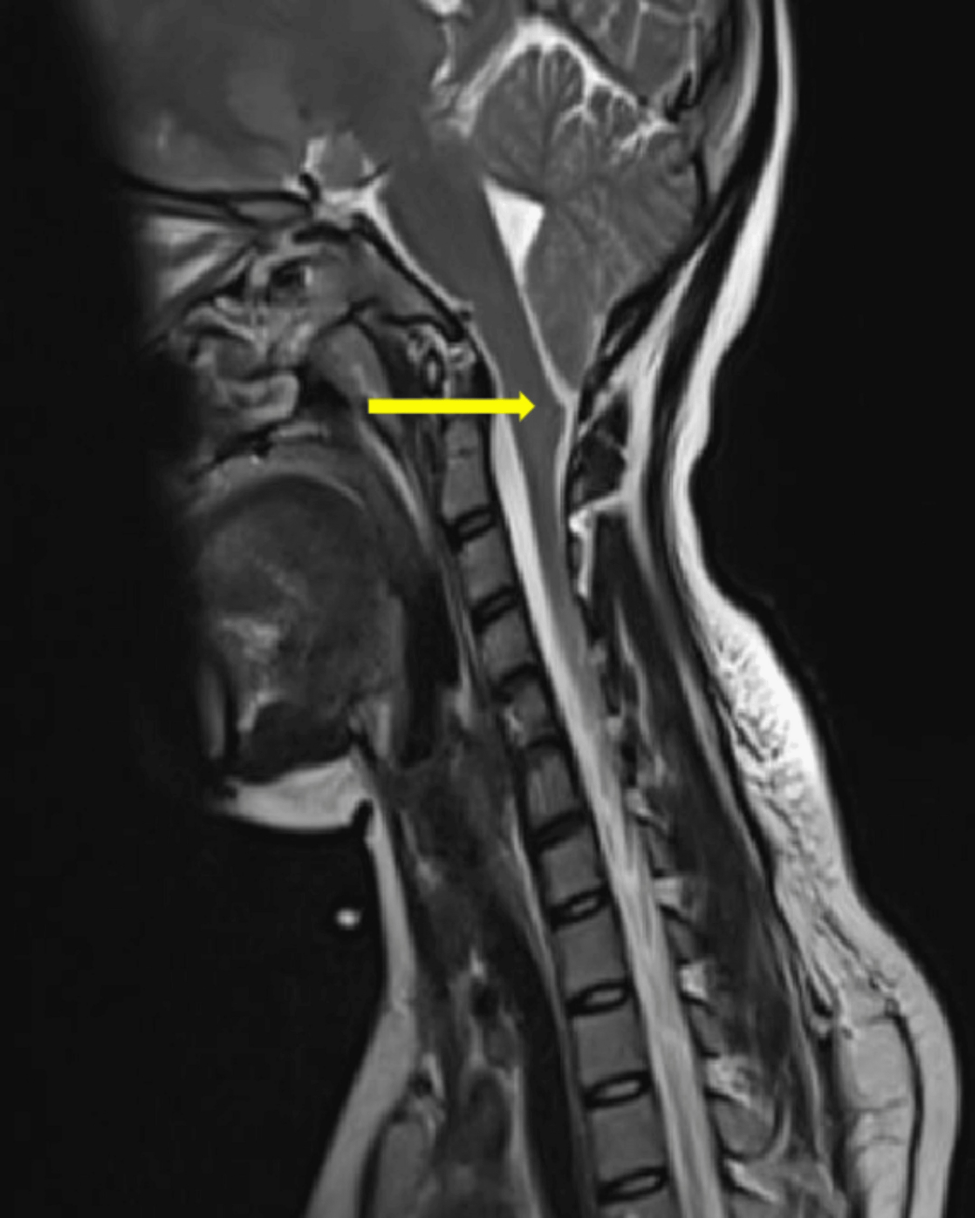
MRI spine T2 Sagittal section: showing Arnold Chiari malformation type 1 (arrow shows the displaced cerebellar tonsils)
